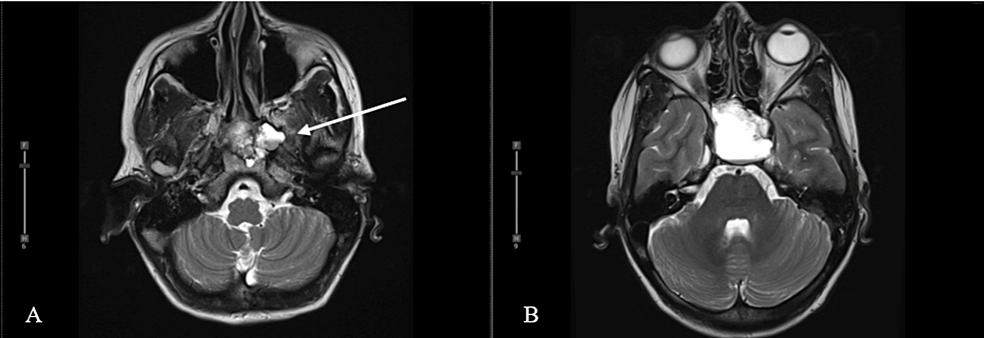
Axial T2-weighted non-contrast magnetic resonance images. Axial T2-weighted non-contrast magnetic resonance images obtained at the level of the masticator space (A) and at the level of the globes (B). Image A demonstrates the asymmetric extension of the lesion through the left sphenoid bone and into the masticator space (arrow). In image B, there is a deformity of the sphenoid sinuses secondary to mass effect from the lesion, greater on the left.
